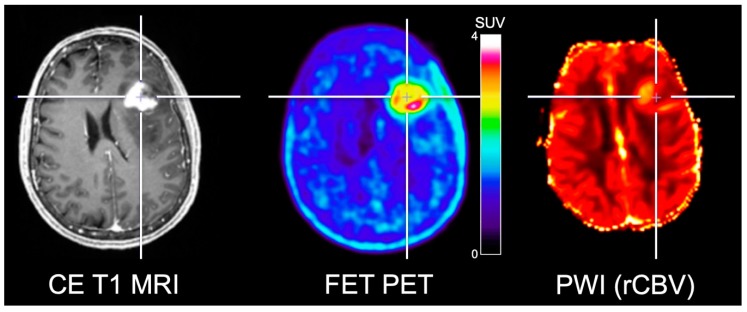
Simultaneously acquired contrast-enhanced (CE) T1-weighted MRI, FET PET and relative cerebral blood volume (rCBV) obtained from PWI in a patient with neuropathologically confirmed glioblastoma. FET PET identifies metabolically active tumor areas without an increased rCBV. Image courtesy of Christian Filss, Institute of Neuroscience and Medicine, Forschungszentrum Juelich, Juelich, Germany.
